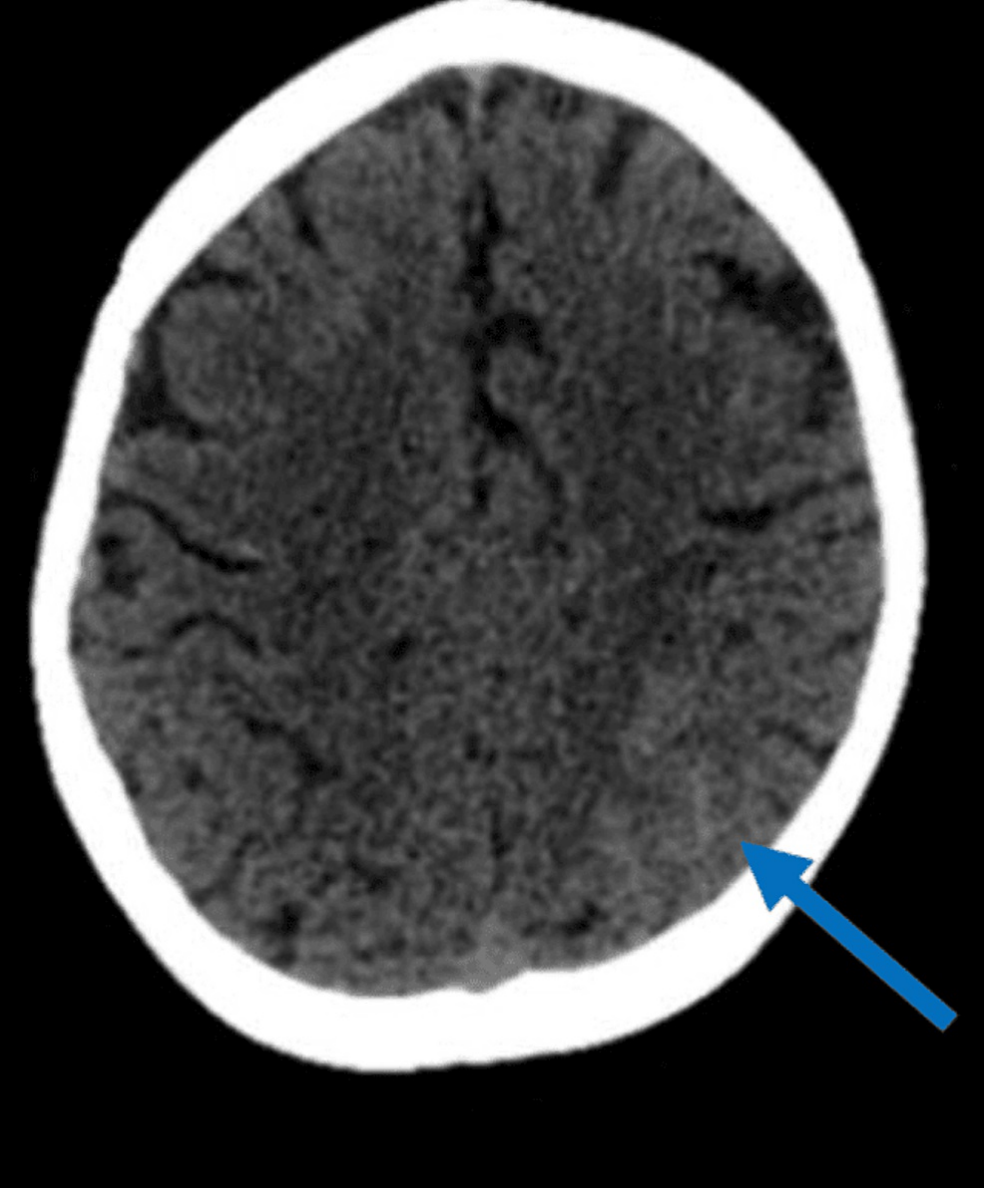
A 45-year-old woman with sudden, severe occipital headache, photophobia, and nausea without any focal neurological deficits. Unenhanced CT shows focal SAH in the left posterior parietal region (blue arrow). CT angiogram (not shown) was normal. CT: computed tomography; SAH: subarachnoid hemorrhage
